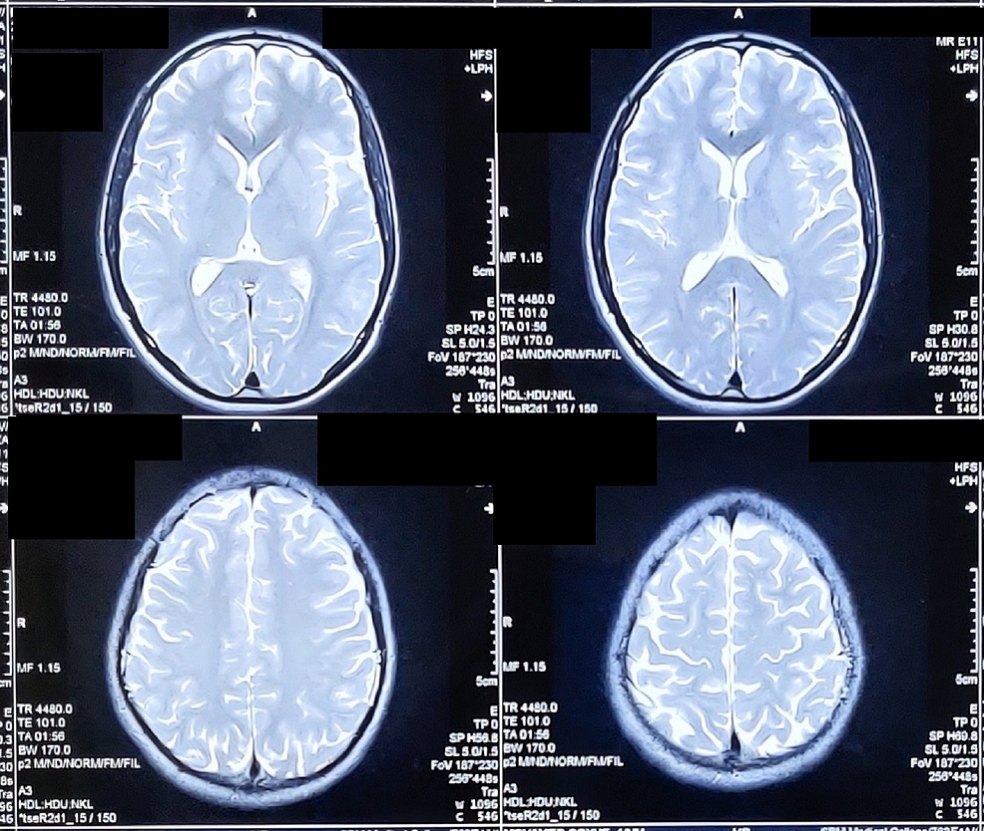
T2-weighted MRI of the brain showing normal study
